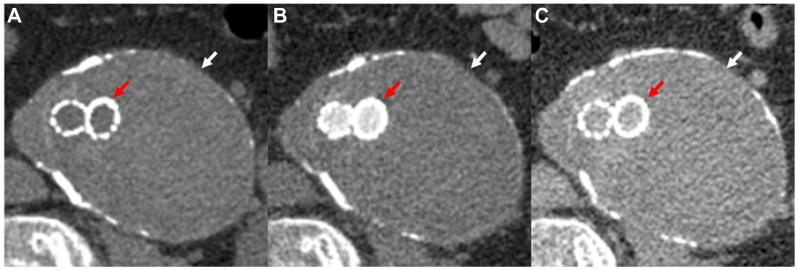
Computed tomography angiography (CTA) with noncontrast (A), arterial (B), and venous (C) phases demonstrating a large abdominal aortic aneurysm (AAA) measuring approximately 7.5 × 9 cm (*white arrow*) with a bifurcated endovascular graft in place (*red arrow*). There was no clear evidence of endoleak.
